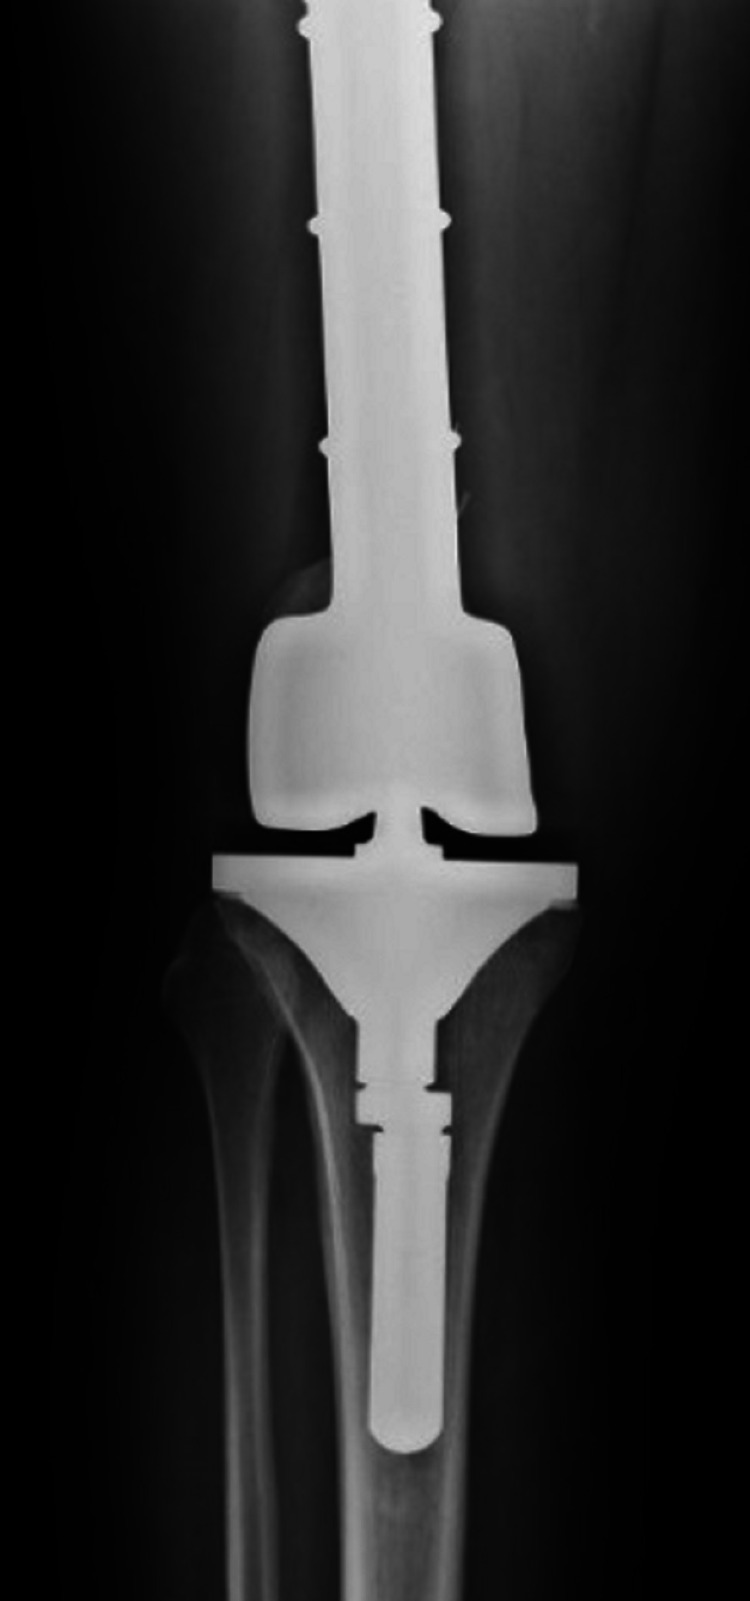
Anteroposterior right knee radiography Knee replacement with prosthetic material
